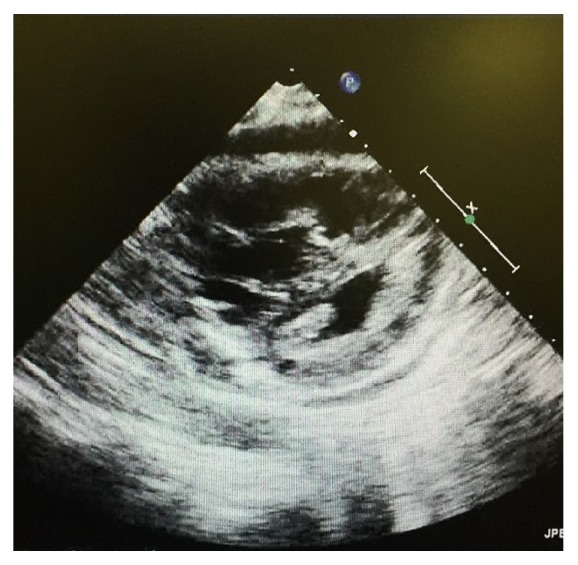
Echocardiogram showing significant dilation of right ventricle along with a slit-like left ventricle.
